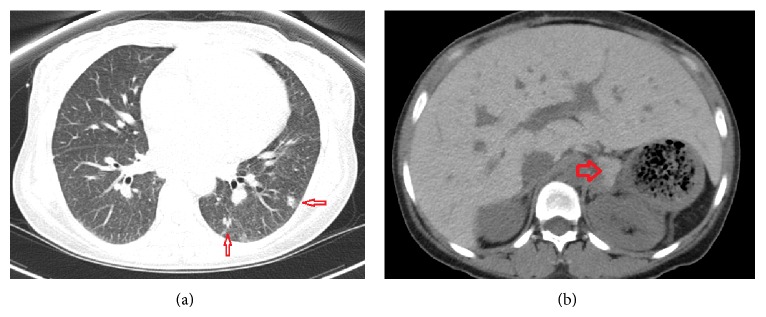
Axial noncontrast CT scan through the lung bases and upper abdomen. (a) Multiple pulmonary nodules are noted in the left lung base (small red arrows). (b) The liver and left adrenal gland (large red arrow) are hyperdense on the noncontrast exam, a finding that is consistent with iron overload.
